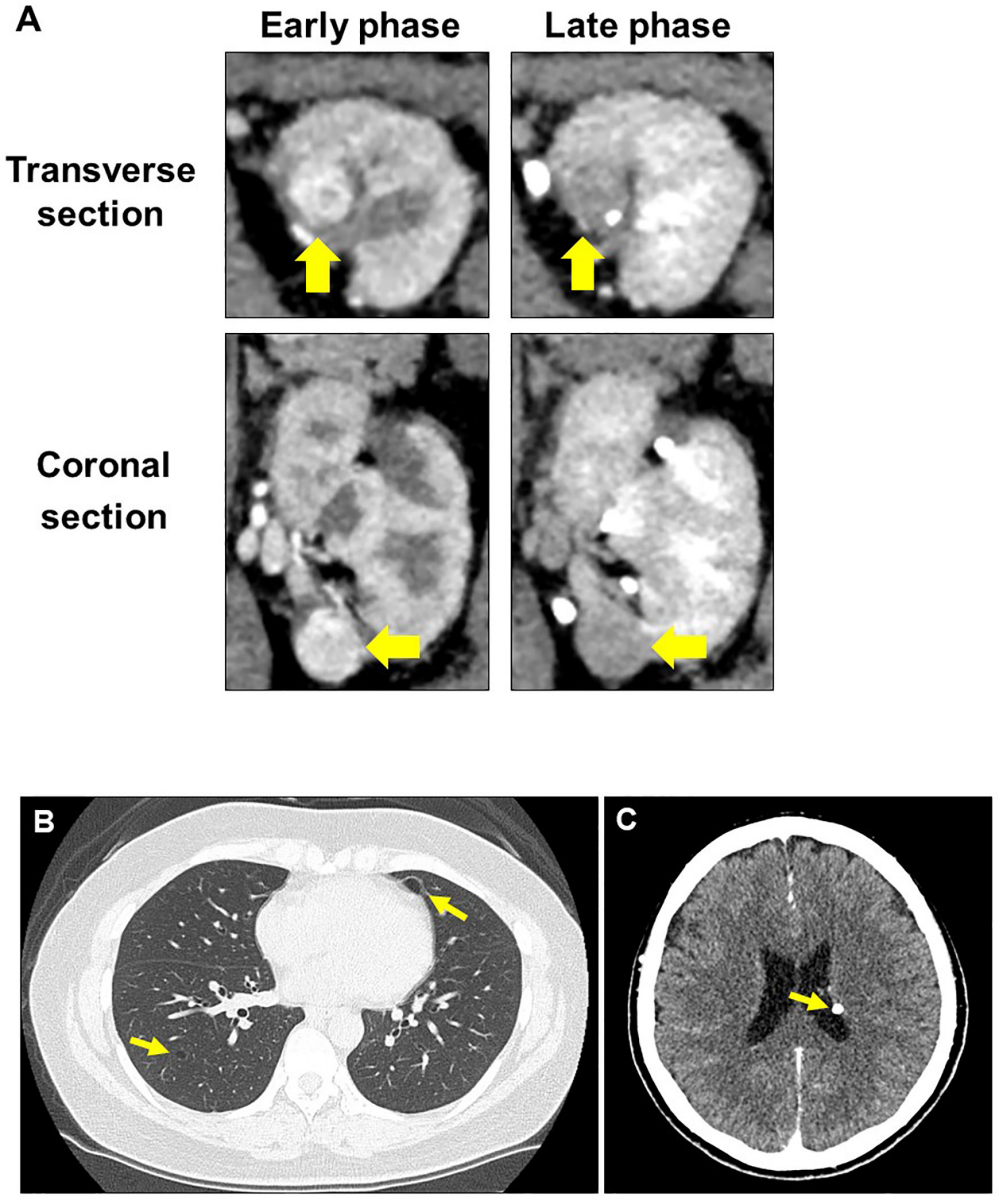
Radiographic evaluation at second recurrence. (A) Computed tomography examination shows typical findings of clear cell renal cell carcinoma in the left kidney. (B) Lung lymphangioleiomyomatosis. (C) Subependymal nodule at the left lateral ventricular wall of the brain.
